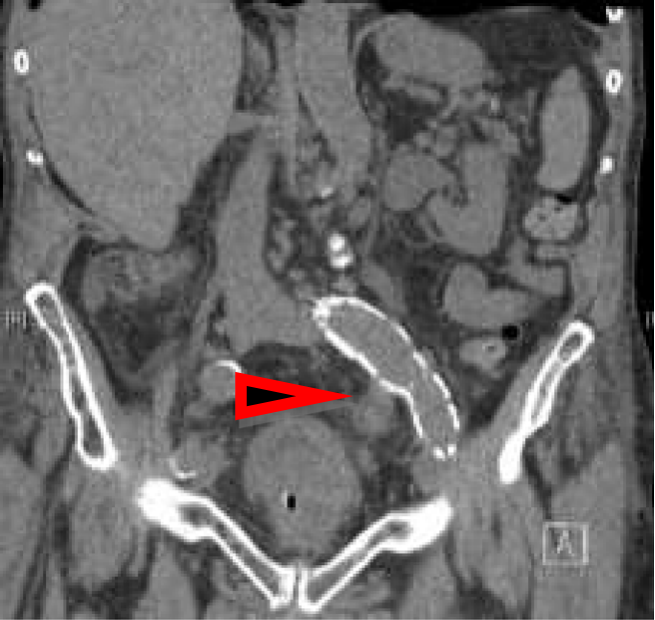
Abdominal CT scan showing the left femoro-popliteal graft and surrounding collection. (See arrow).
